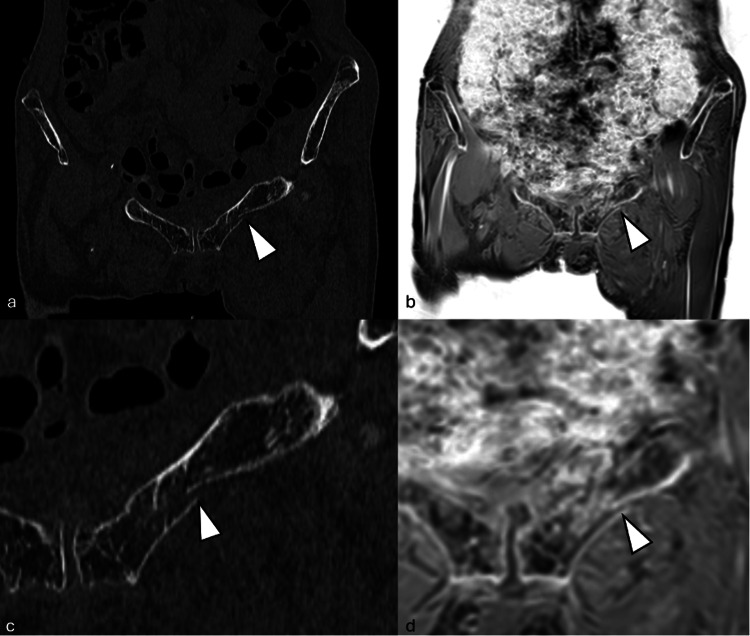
Representative imaging example 1. A patient with a left superior pubic ramus fracture is shown in (a, c) CT and in (b, d) pseudo-CT. The cortical break was clearly visualized and with high resemblance on both modalities (arrowheads).
